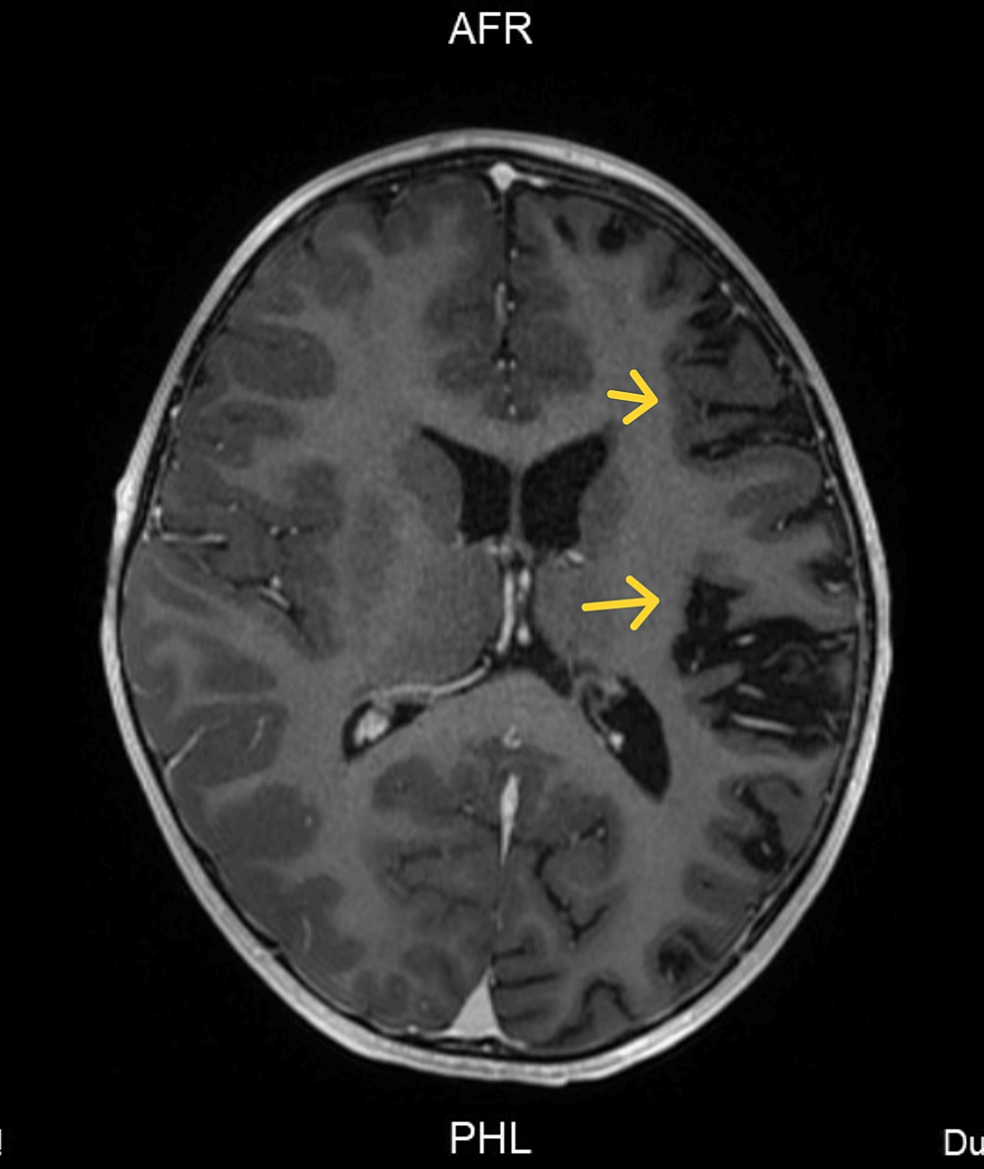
Second brain MRI with contrast axial section Non-enhancement observed on the left hemisphere.
